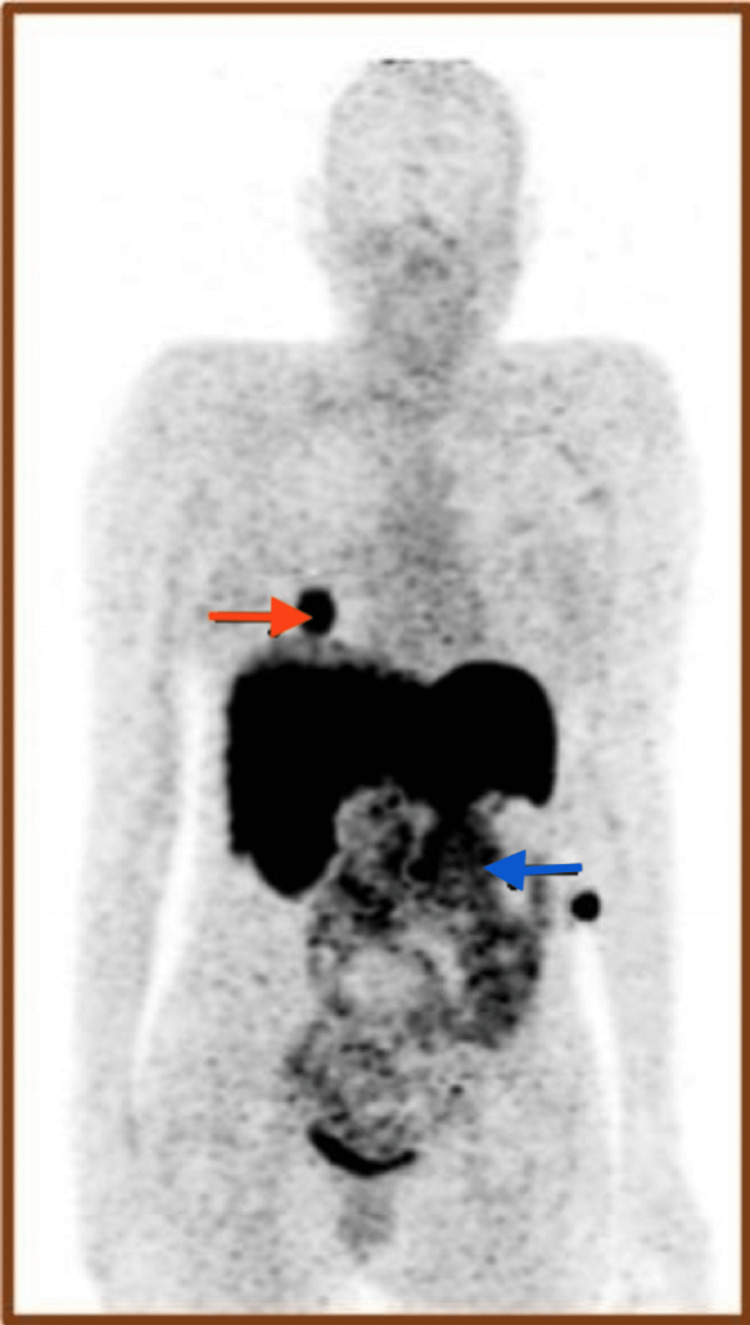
68Ga-DOTANOC whole-body PET/CT Showing increased radiotracer uptake in the left paraaortic region (blue arrow) and a metastatic nodule in the right lung lower lobe (red arrow) before surgery
